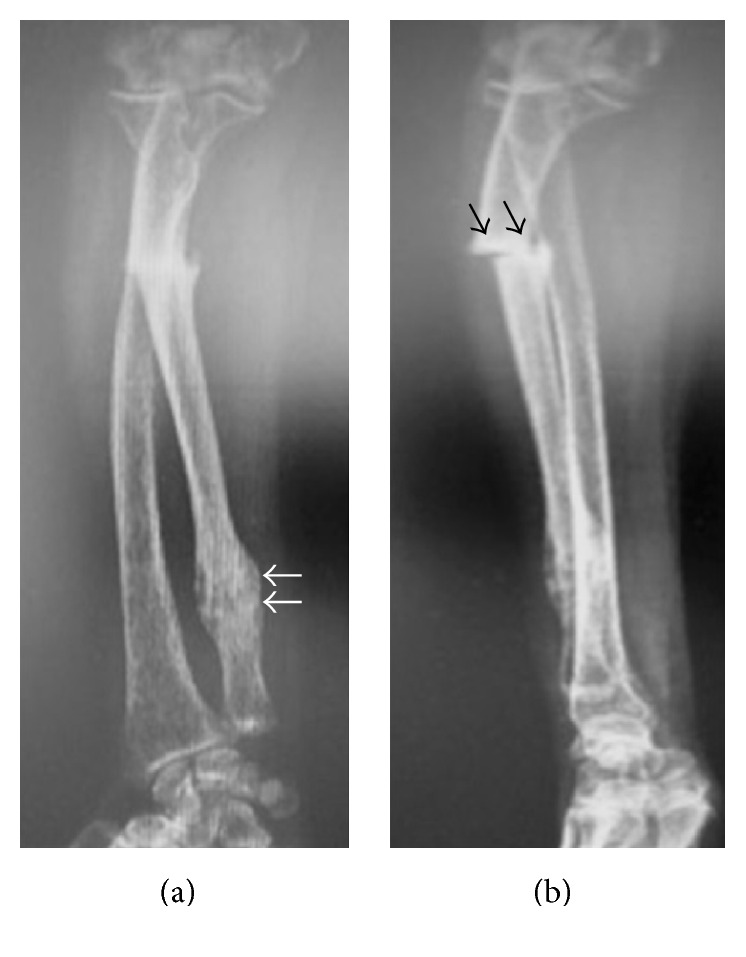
Anteroposterior (a) and lateral radiographs (b) of the patient's forearm at initial admission. Black arrows show the atypical fracture line. White arrows show the previous healed fracture.
